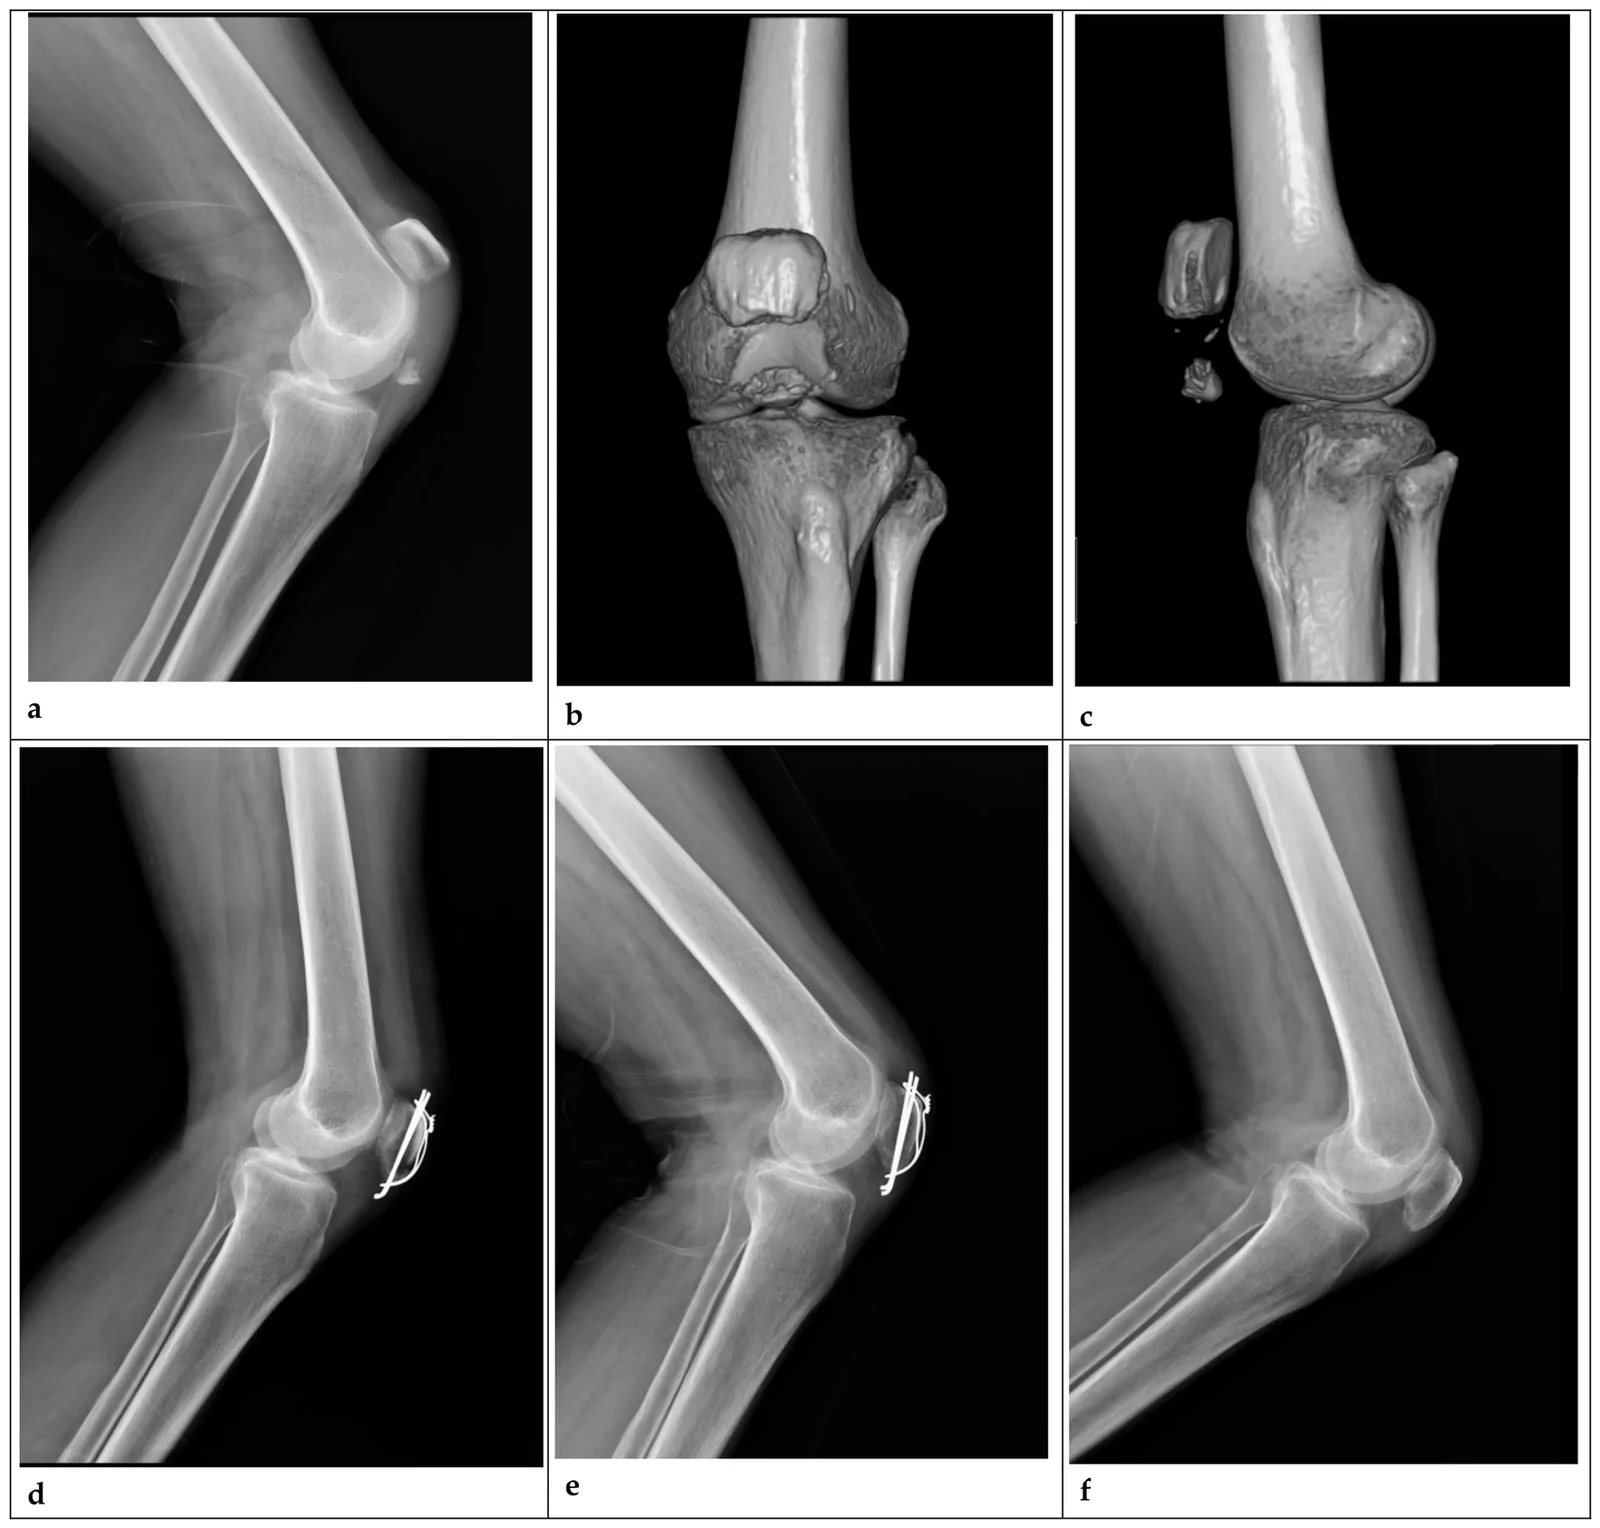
Preoperative and postoperative imaging of a left inferior patellar pole fracture. (a–c) Preoperative lateral radiographs and three-dimensional computed tomography (CT) images demonstrate a displaced fracture of the left inferior patellar pole. (d) The immediate postoperative lateral radiograph shows satisfactory fracture reduction and appropriate positioning of the internal fixation. (e) At the 1-year follow-up, the lateral radiograph reveals solid bone union with no evidence of implant loosening. (f) After removal of the internal fixation, the lateral radiograph confirms solid bone union.
